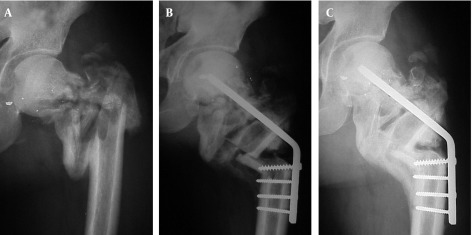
A. Bullet Injury Resulting in SevereComminution of Upper Left Femur and Coxa Vara. B. Post-operative X-ray After Wedge Removing Valgus Osteotomy Below the Non-union Mass and 130° Plate Fixation. C. Follow-up, Five Months Later Showing a Solid Bony Mass Uniting the Femoral Neck Fracture and the Osteotomy.
